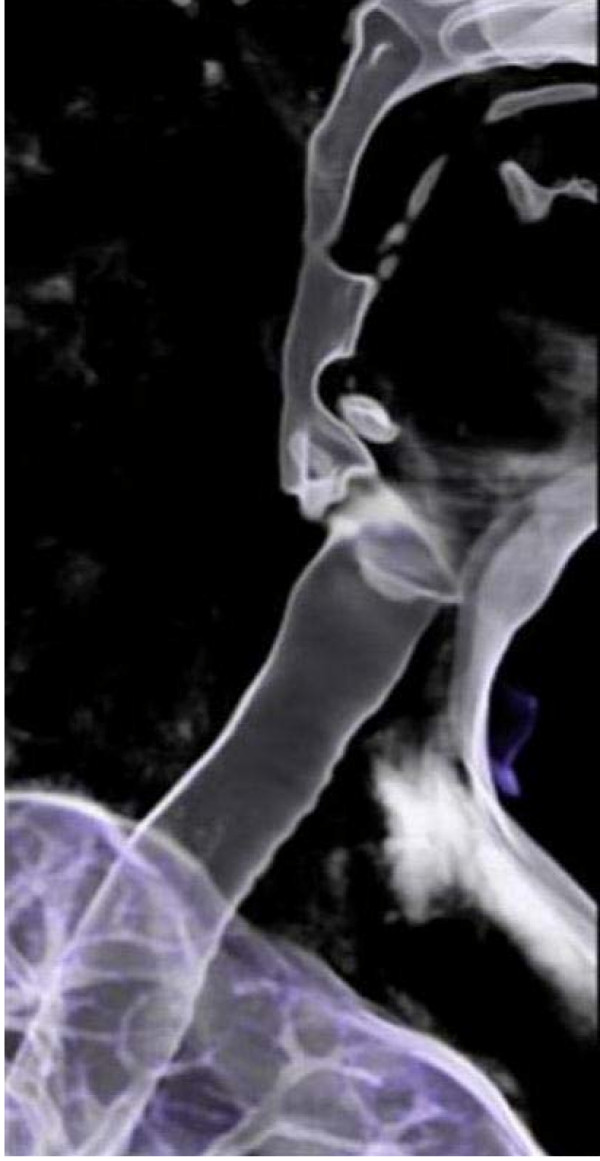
A facial computed tomography reformation at 5.5 months after the initial presentation shows an angular dysmorphia at the hypopharynx level.
